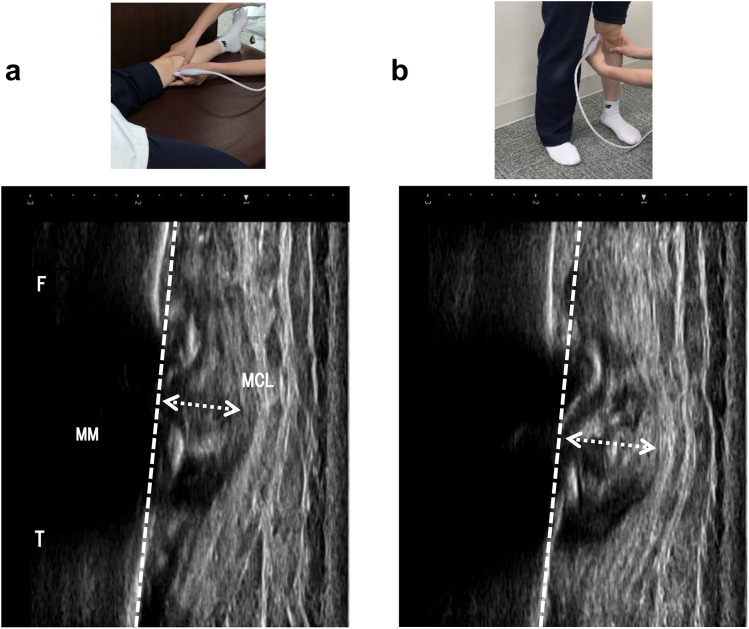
The positions of ultrasound assessment and corresponding images. The ultrasound images of medial meniscus extrusion in nonweight-bearing (a) and weight-bearing (b). MM, medial meniscus; F, femur, T, tibia, MCL, medial collateral ligament. The dashed lines and white double arrows show the line of the medial tibial plateau cortex and the distance of meniscus extrusion.
